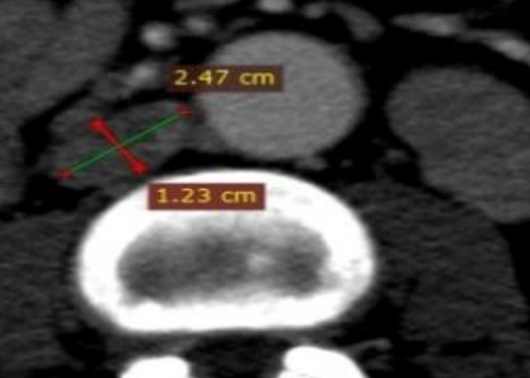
Transverse slice from computed tomography angiography showing the inferior vena cava measuring 24.7 mm in its largest diameter, 3 weeks after surgery.
